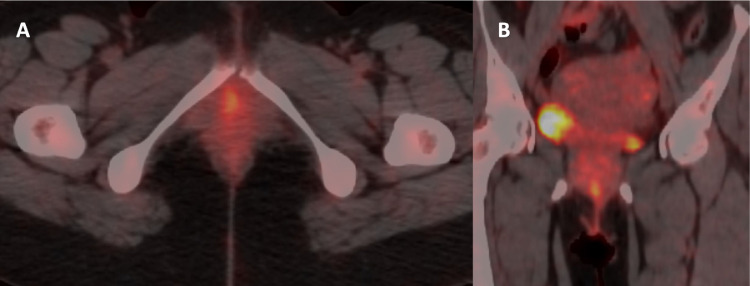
FDG PET CT scan of a 39-year-old female, presenting with rapidly enlarging 1 cm vulval lump, shows FDG-avid metabolically active lesion involving right vulva without invasion of the urethra, bone or muscle. June 2019, preoperative. (A, axial view; B, coronal view).
